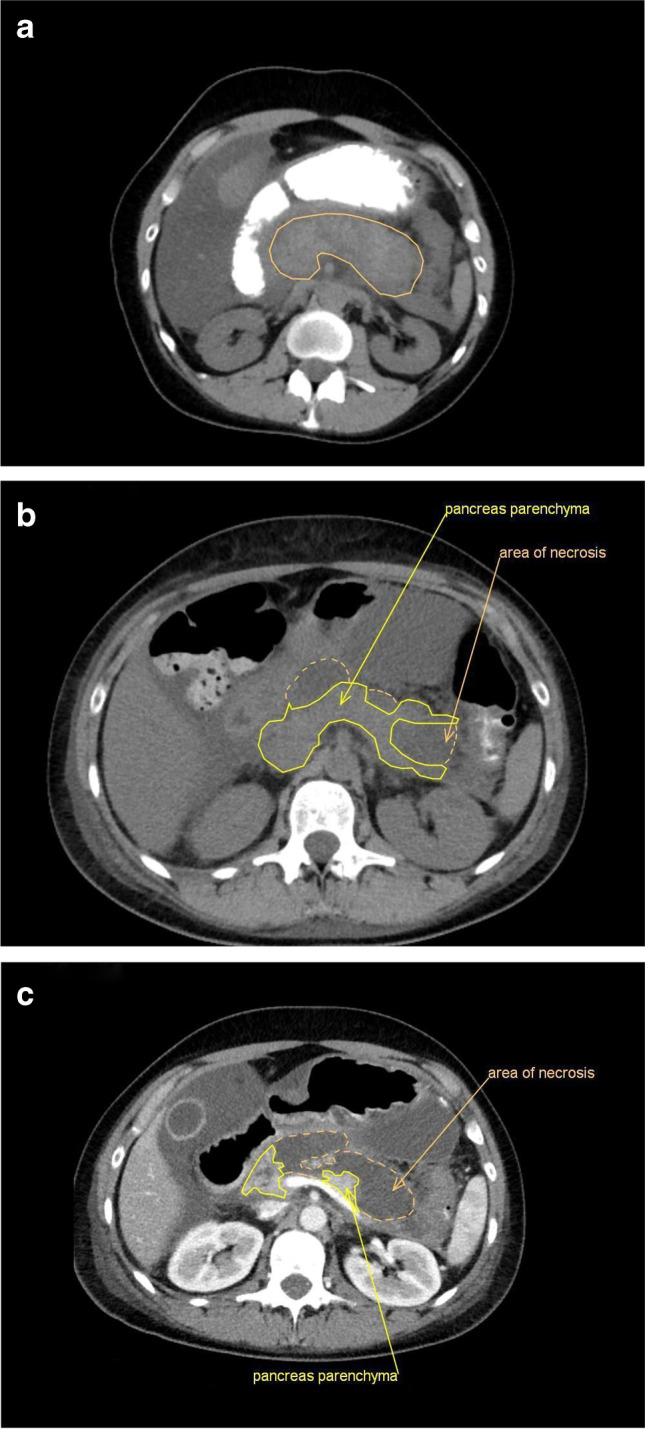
a Native abdominal CT scan with oral contrast dye at the day of admission: acute edematous pancreatitis (border of the pancreas represented by solid line) with significant reaction of the surrounding tissue representing beginning necrotic streets. b Native abdominal CT scan 8 days later: no clear signs of pancreatic necrosis (dashed line) with ongoing strong necrotic streets. c Abdominal CT scan after intravenous contrast dye another 2 days later: farther pronounced and progressive necrotic streets, increasing pancreas necrosis with less and less normal parenchyma left
